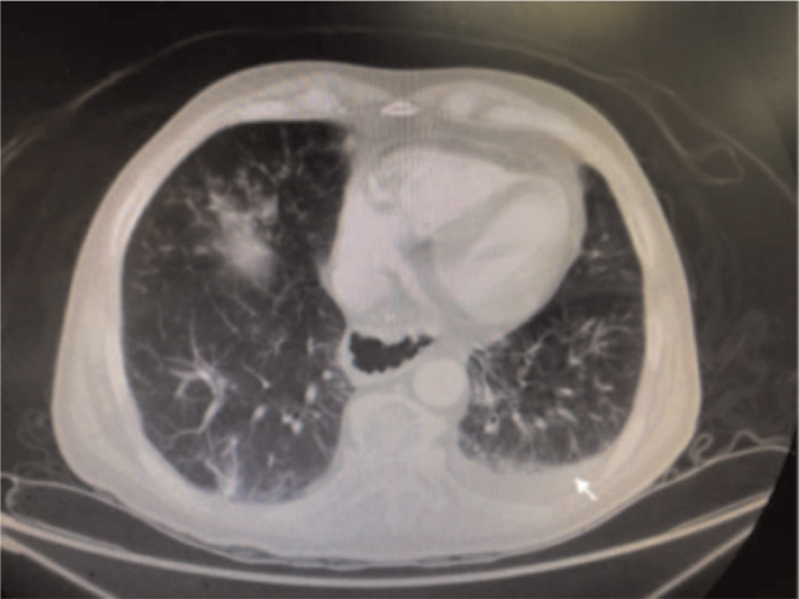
Computed tomography of the chest shows infiltration of the left lower lobe with pleural effusion, indicated by white arrow.
